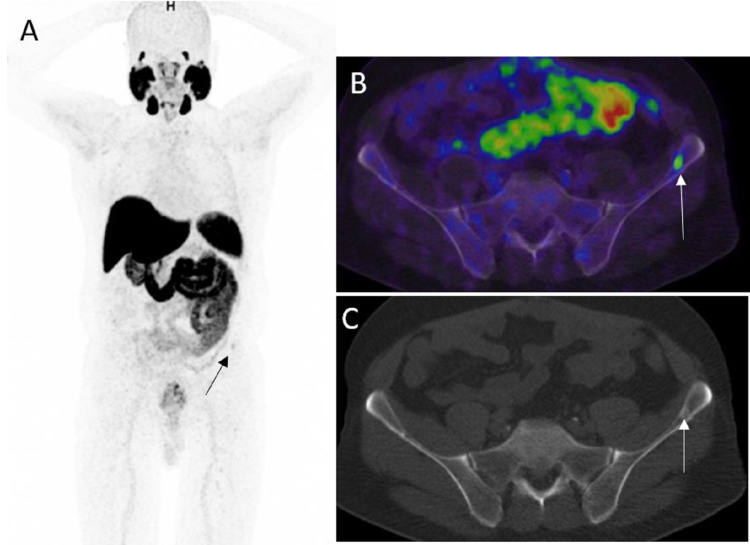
PSMA-RADS-3B A: 68Ga PSMA PET MIP image shows a single focus of abnormal uptake in the left ilium (black arrow). B: 68Ga PSMA PET/CT axial image through the pelvis shows small focal radiotracer uptake in the left ilium (white arrow). C: CT axial image through the pelvis in the same patient shows the correlate small indeterminate sclerotic lesion in the left ilium (white arrow).
